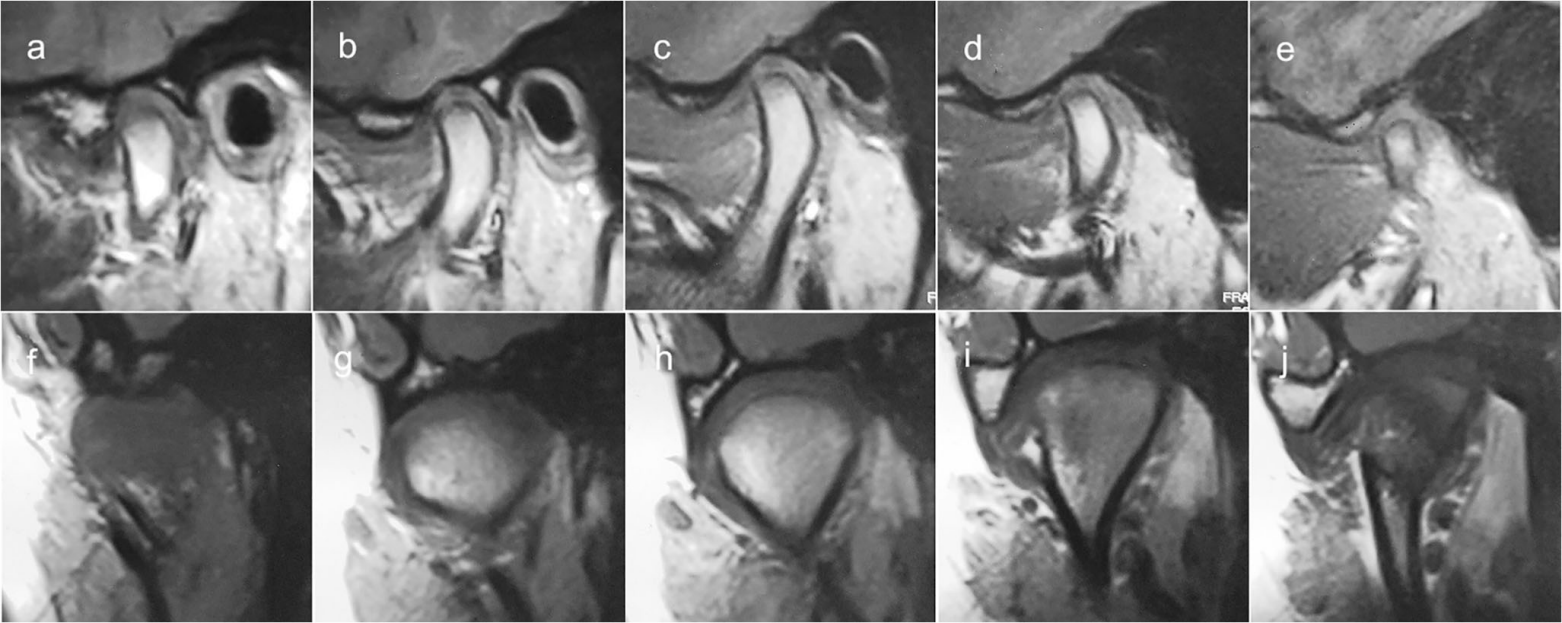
MRI of TMJ with lateral disc displacement. a–e Oblique sagittal slices in intercuspal position. f–j Oblique coronal slices in intercuspal position
